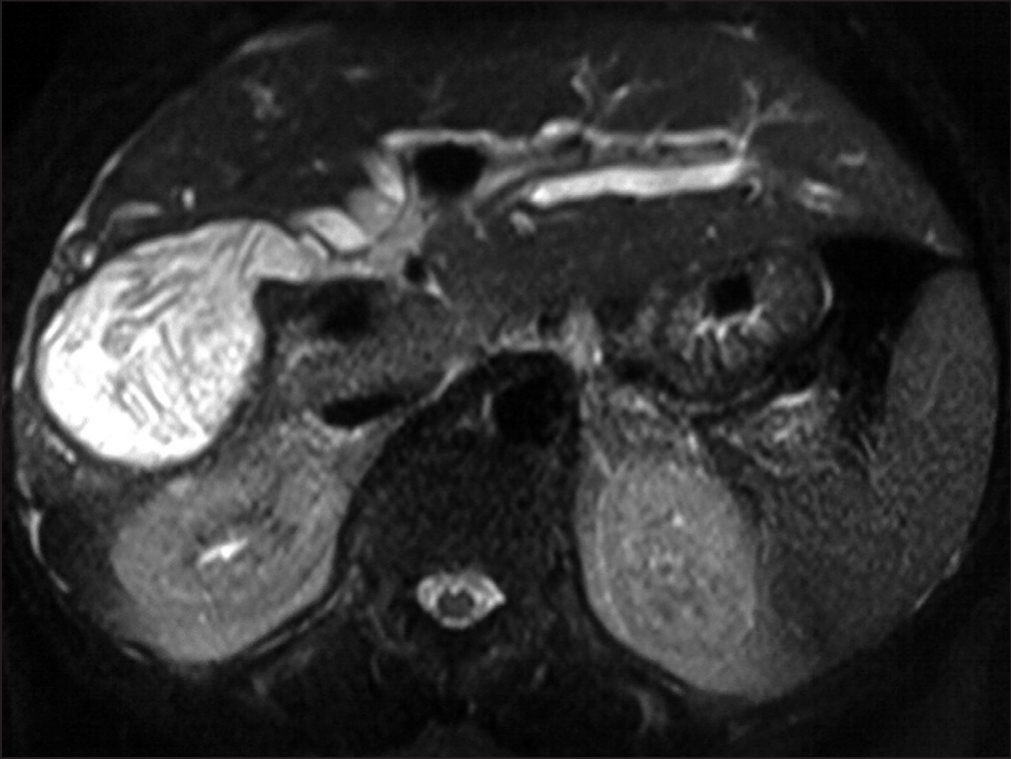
Axial T2W MR image showing a cyst in the right lobe of liver posteriorly; medial aspect of the cyst shows a beak like projection from the wall into the right posterior sectoral duct. Membranes are seen within the cyst
